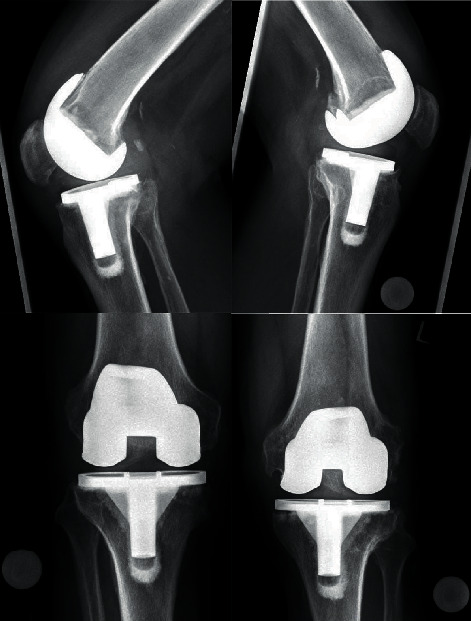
The preoperative diagnostics included radiographs of both knees in 2 planes.
